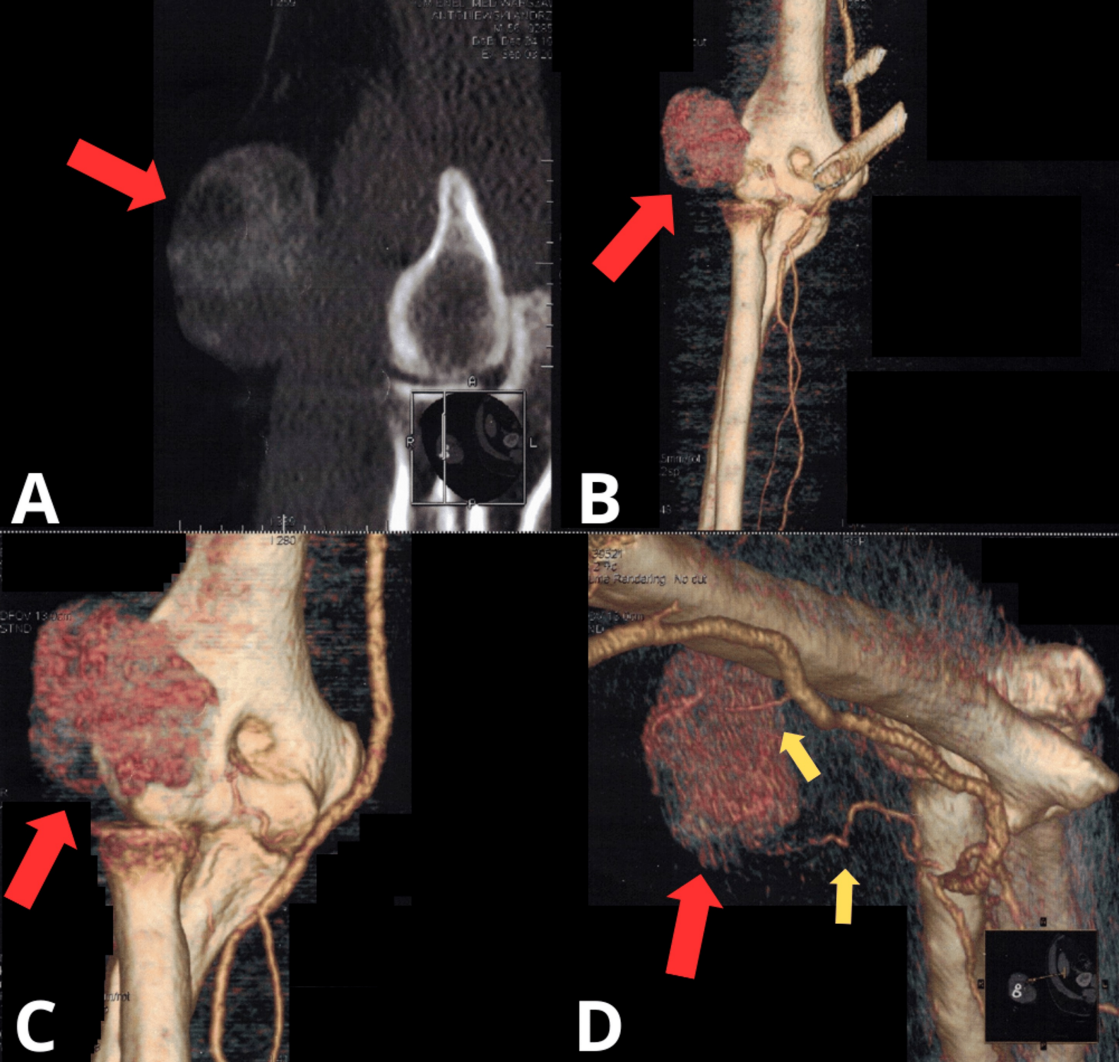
Imaging diagnostics of the tumor mass on CT CT: Computed Tomography; 2D: Two-dimensional; 3D: Three-dimensional (A) Axial 2D CT view showing the tumor mass; (B and C) 3D reconstruction of the elbow joint, anterior projection; (D) 3D reconstruction of the elbow joint, lateral projection. Red arrow indicates the tumor mass and the yellow arrow indicates the feeding vessels supplying the tumor.
